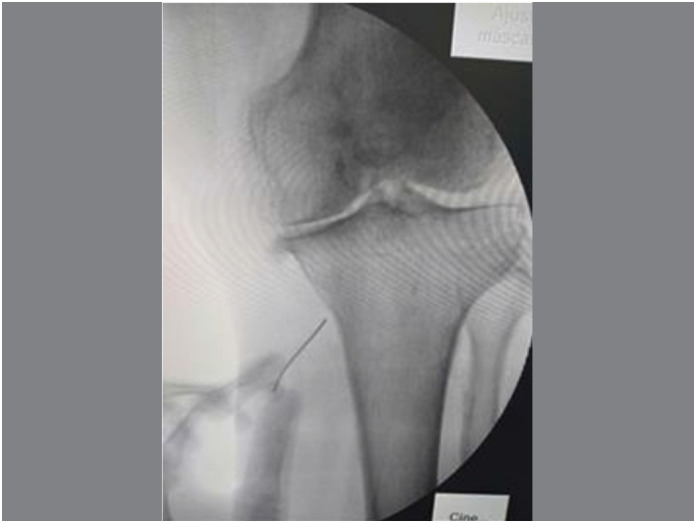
Positioning in AP incidence for thermoregulation of the inferior medial genicular nerve. AP x-ray incidence of medial inferior genicular nerve radiofrequency.
